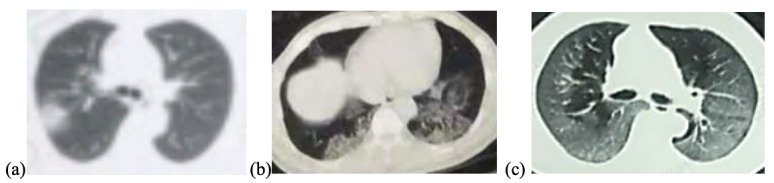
Lesion distribution on computed tomography (CT) images in patients with coronavirus disease pneumonia. (a) 60-year-old woman, unilateral lesion distribution; the axial CT image shows patchy shadowing in the right upper lobe. This patient recovered after 20 days of treatment in hospital. (b) 53-year-old man, bilateral lesion distribution; the axial CT image shows mixed lesions with ground-glass opacity and patchy shadowing in the bilateral lower lobes. This patient recovered after 30 days of treatment in hospital. (c) 68-year-old man, diffuse lesion distribution; the axial CT image shows ground-glass opacity in bilateral lungs. This patient died without treatment in hospital.
